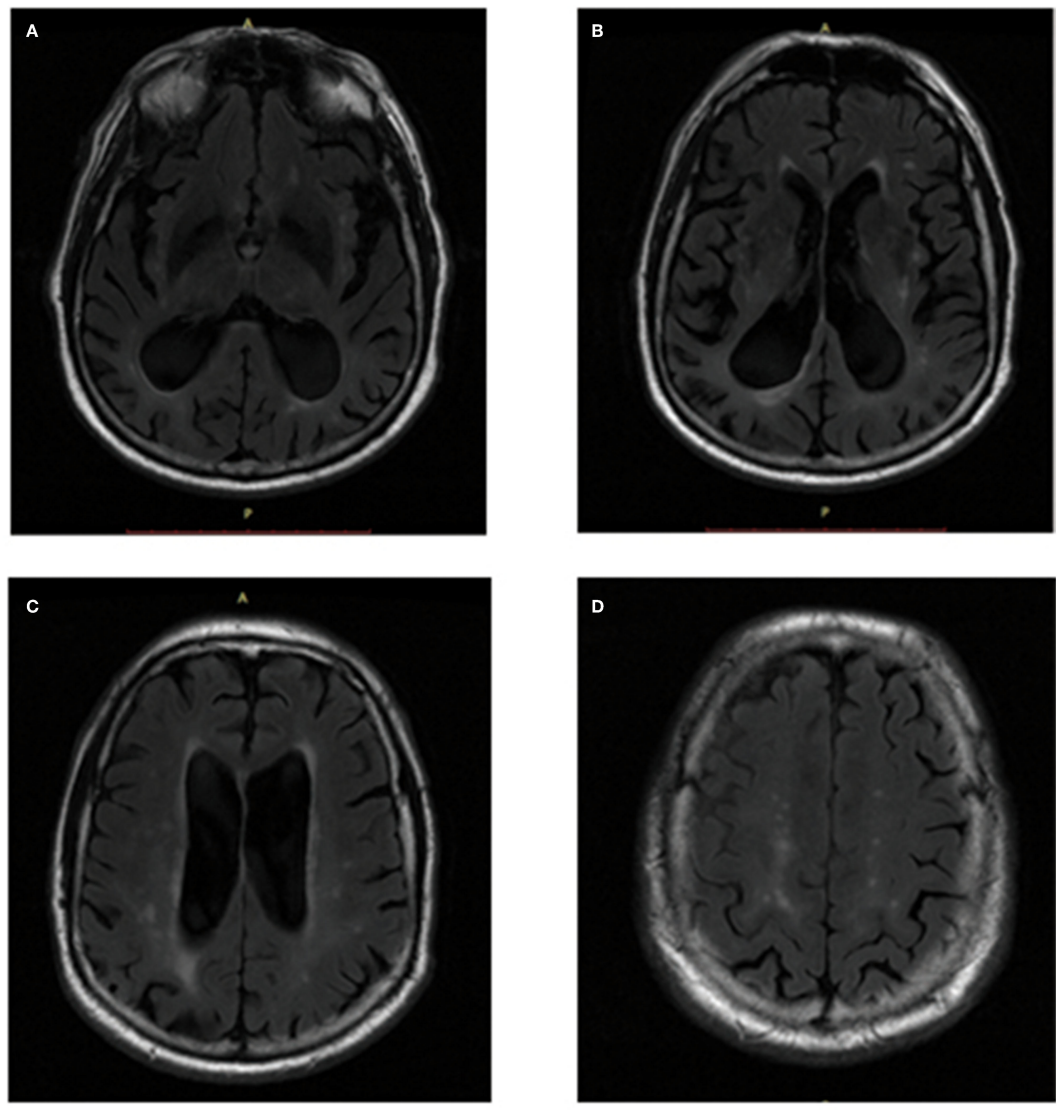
CHIPS scores showed in T2-FLAIR images. (A) Low External Capsule: Anterior (Right = 0, Left = 1, Factor = 4, Total = 4); Posterior (Right = 1, Left = 1, Factor = 4, Total = 8). (B) High External Capsule: EC Anterior (Right = 1, Left = 1, Factor = 3, Total = 6); EC Posterior (Right = 1, Left = 1, Factor = 3, Total = 6); Cingulate (Right = 0, Left = 0, Factor = 4, Total = 0). (C) Corona Radiata: CR Anterior (Right = 1, Left = 1, Factor = 2, Total = 4); CR Posterior (Right = 1, Left = 1, Factor = 2, Total = 4); Cingulate (Right = 0, Left = 0, Factor = 1, Total = 0). (D) Centrum Semiovale: Anterior (Right = 0, Left = 1, Factor = 1, Total = 1); Posterior (Right = 1, Left = 1, Factor = 1, Total = 2).
